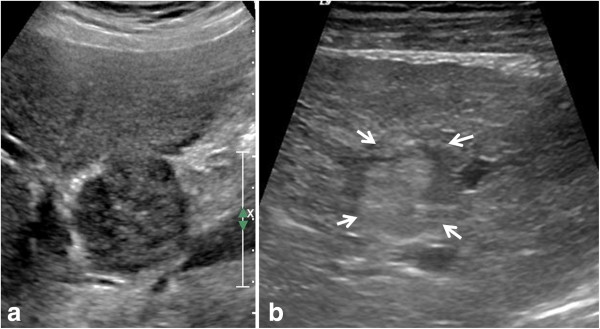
Examples of intrahepatic cholangiocarcinoma (iCCA) on ultrasound in two different patients. Mass forming iCCA may present as a well-defined hypoechoic mass (arrow, a) or as an ill-defined heterogeneous isoechoic mass (arrowheads, b).
